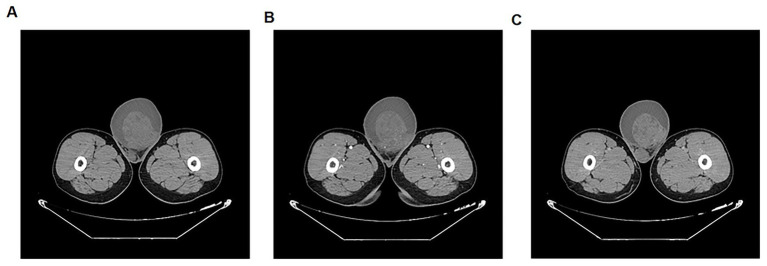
The conventional plain scan (without contrast agent injection) (A), arterial phase (B), and venous phase (C) images of the testicular enhanced CT examination.
